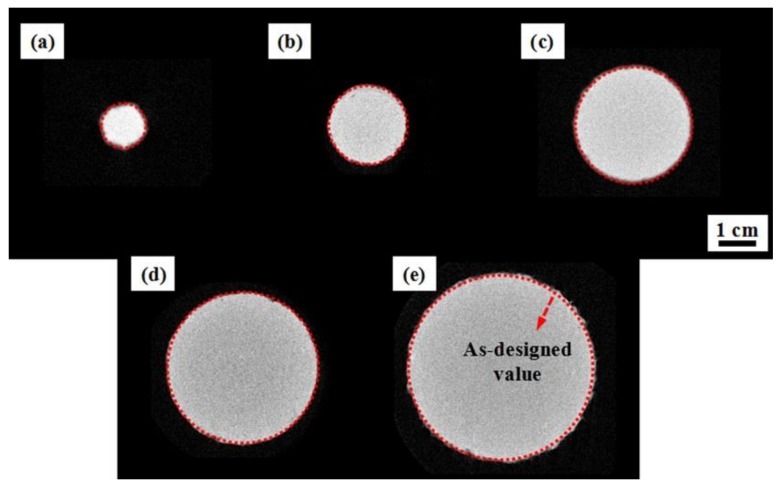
Two-dimensional (2D) segmented CT slices of cross-sections of AlSi10Mg samples for different build sizes: (a) 1 mm; (b) 2 mm; (c) 3 mm; (d) 4 mm and (e) 5 mm. (Red dotted circles indicate the design values).
